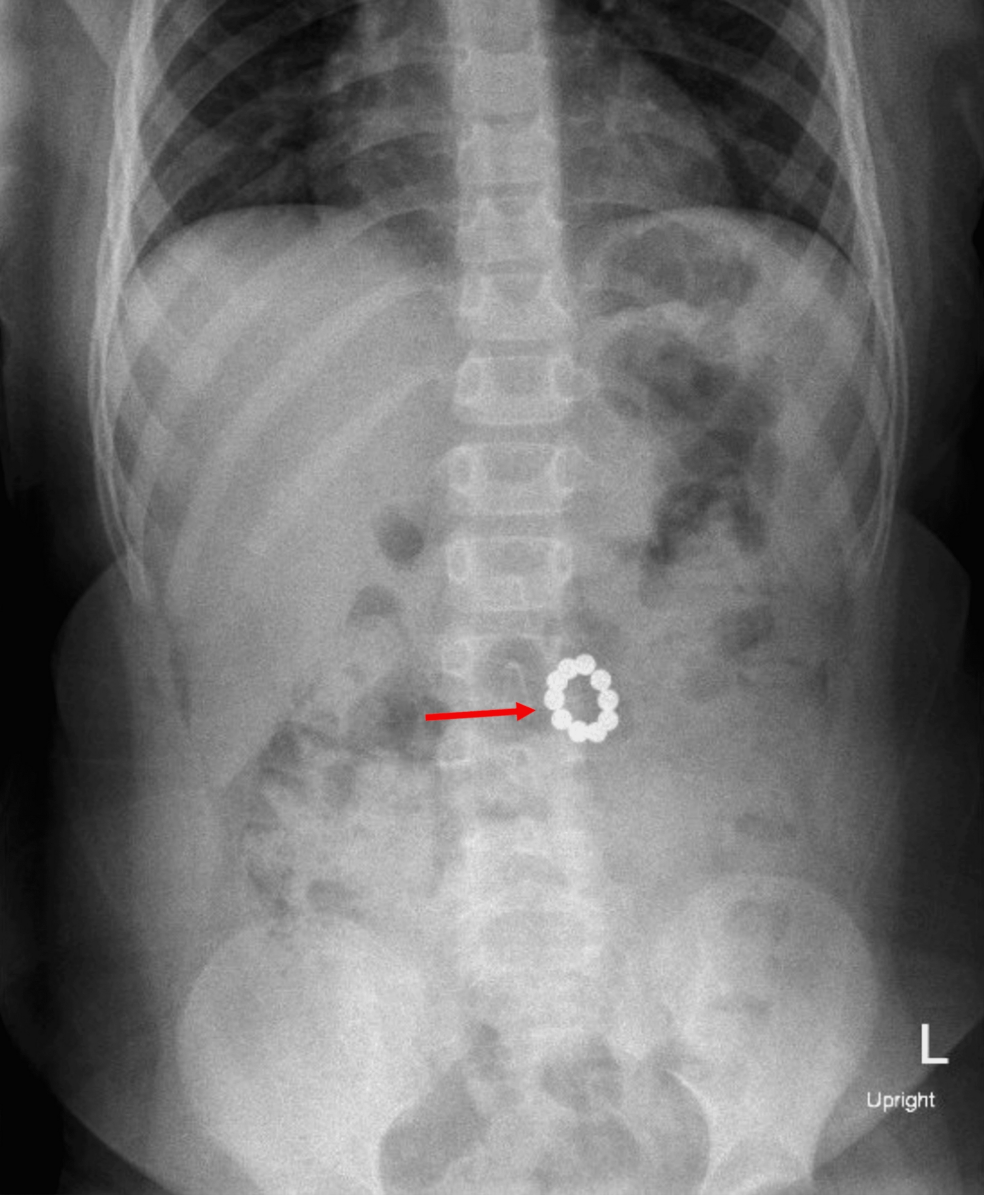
X-ray demonstrating the formation of a "signet ring" (red marker) in the bowel after ingestion of multiple magnets
